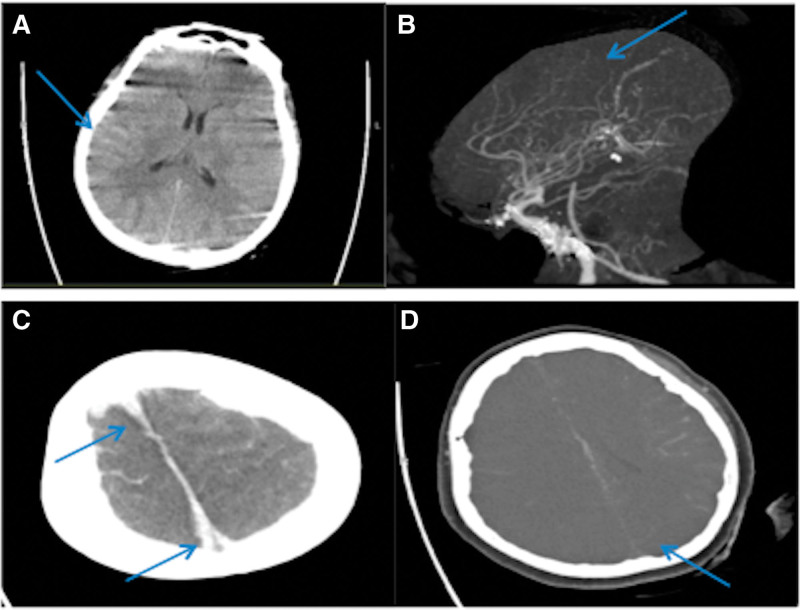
(A) Brain CT showed subarachnoid hemorrhage. (B) Brain CTA showed that no obvious dilatation, stenosis, or tumor-like lesions were found. In the cerebral artery circle, anterior cerebral arteries, middle cerebral arteries, and posterior cerebral arteries on both sides and their branches. Superior sagittal sinus and its surrounding veins were poorly displayed, so superior sagittal sinus thrombosis was considered. (C) Brain CTV showed the density of the superior sagittal sinus increased and brain edema occurred on CT scan. Superior sagittal sinus thrombosis was considered. (D) Brain CTV showed poor development of intracranial veins. CT = computed tomography, CTA = computed tomographic angiography, CTV = computed tomographic venography.
